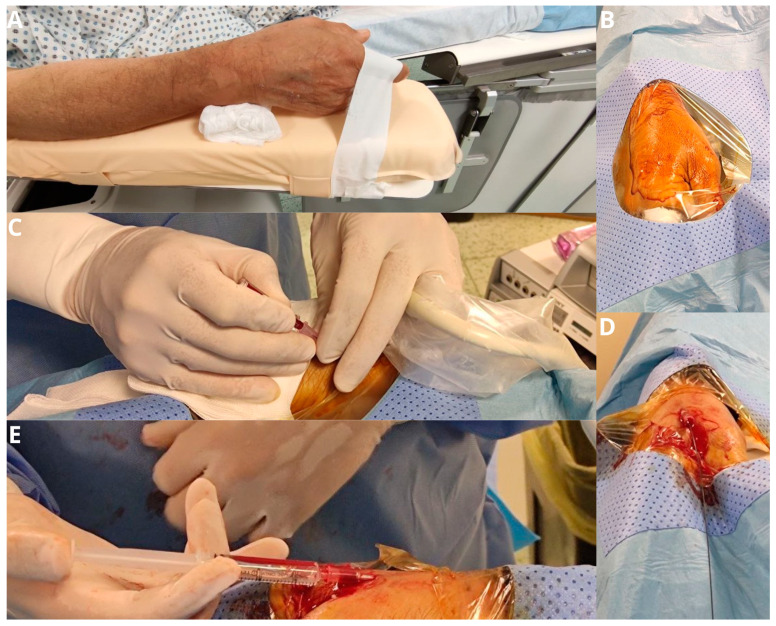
The patient’s forearm is positioned in a neutral semi-pronated position (A) and a drape is placed over the hand after it is prepped, exposing the anatomical snuff box (B). Arterial puncture of the distal radial artery is performed with a 21-gauge micro-puncture needle under ultrasound guidance (C). The wire is advanced through the needle in the vessel in a retrograde fashion (D). If there is any resistance in passing the wire further in the forearm, further imaging with fluoroscopy and angiography is performed (E).
